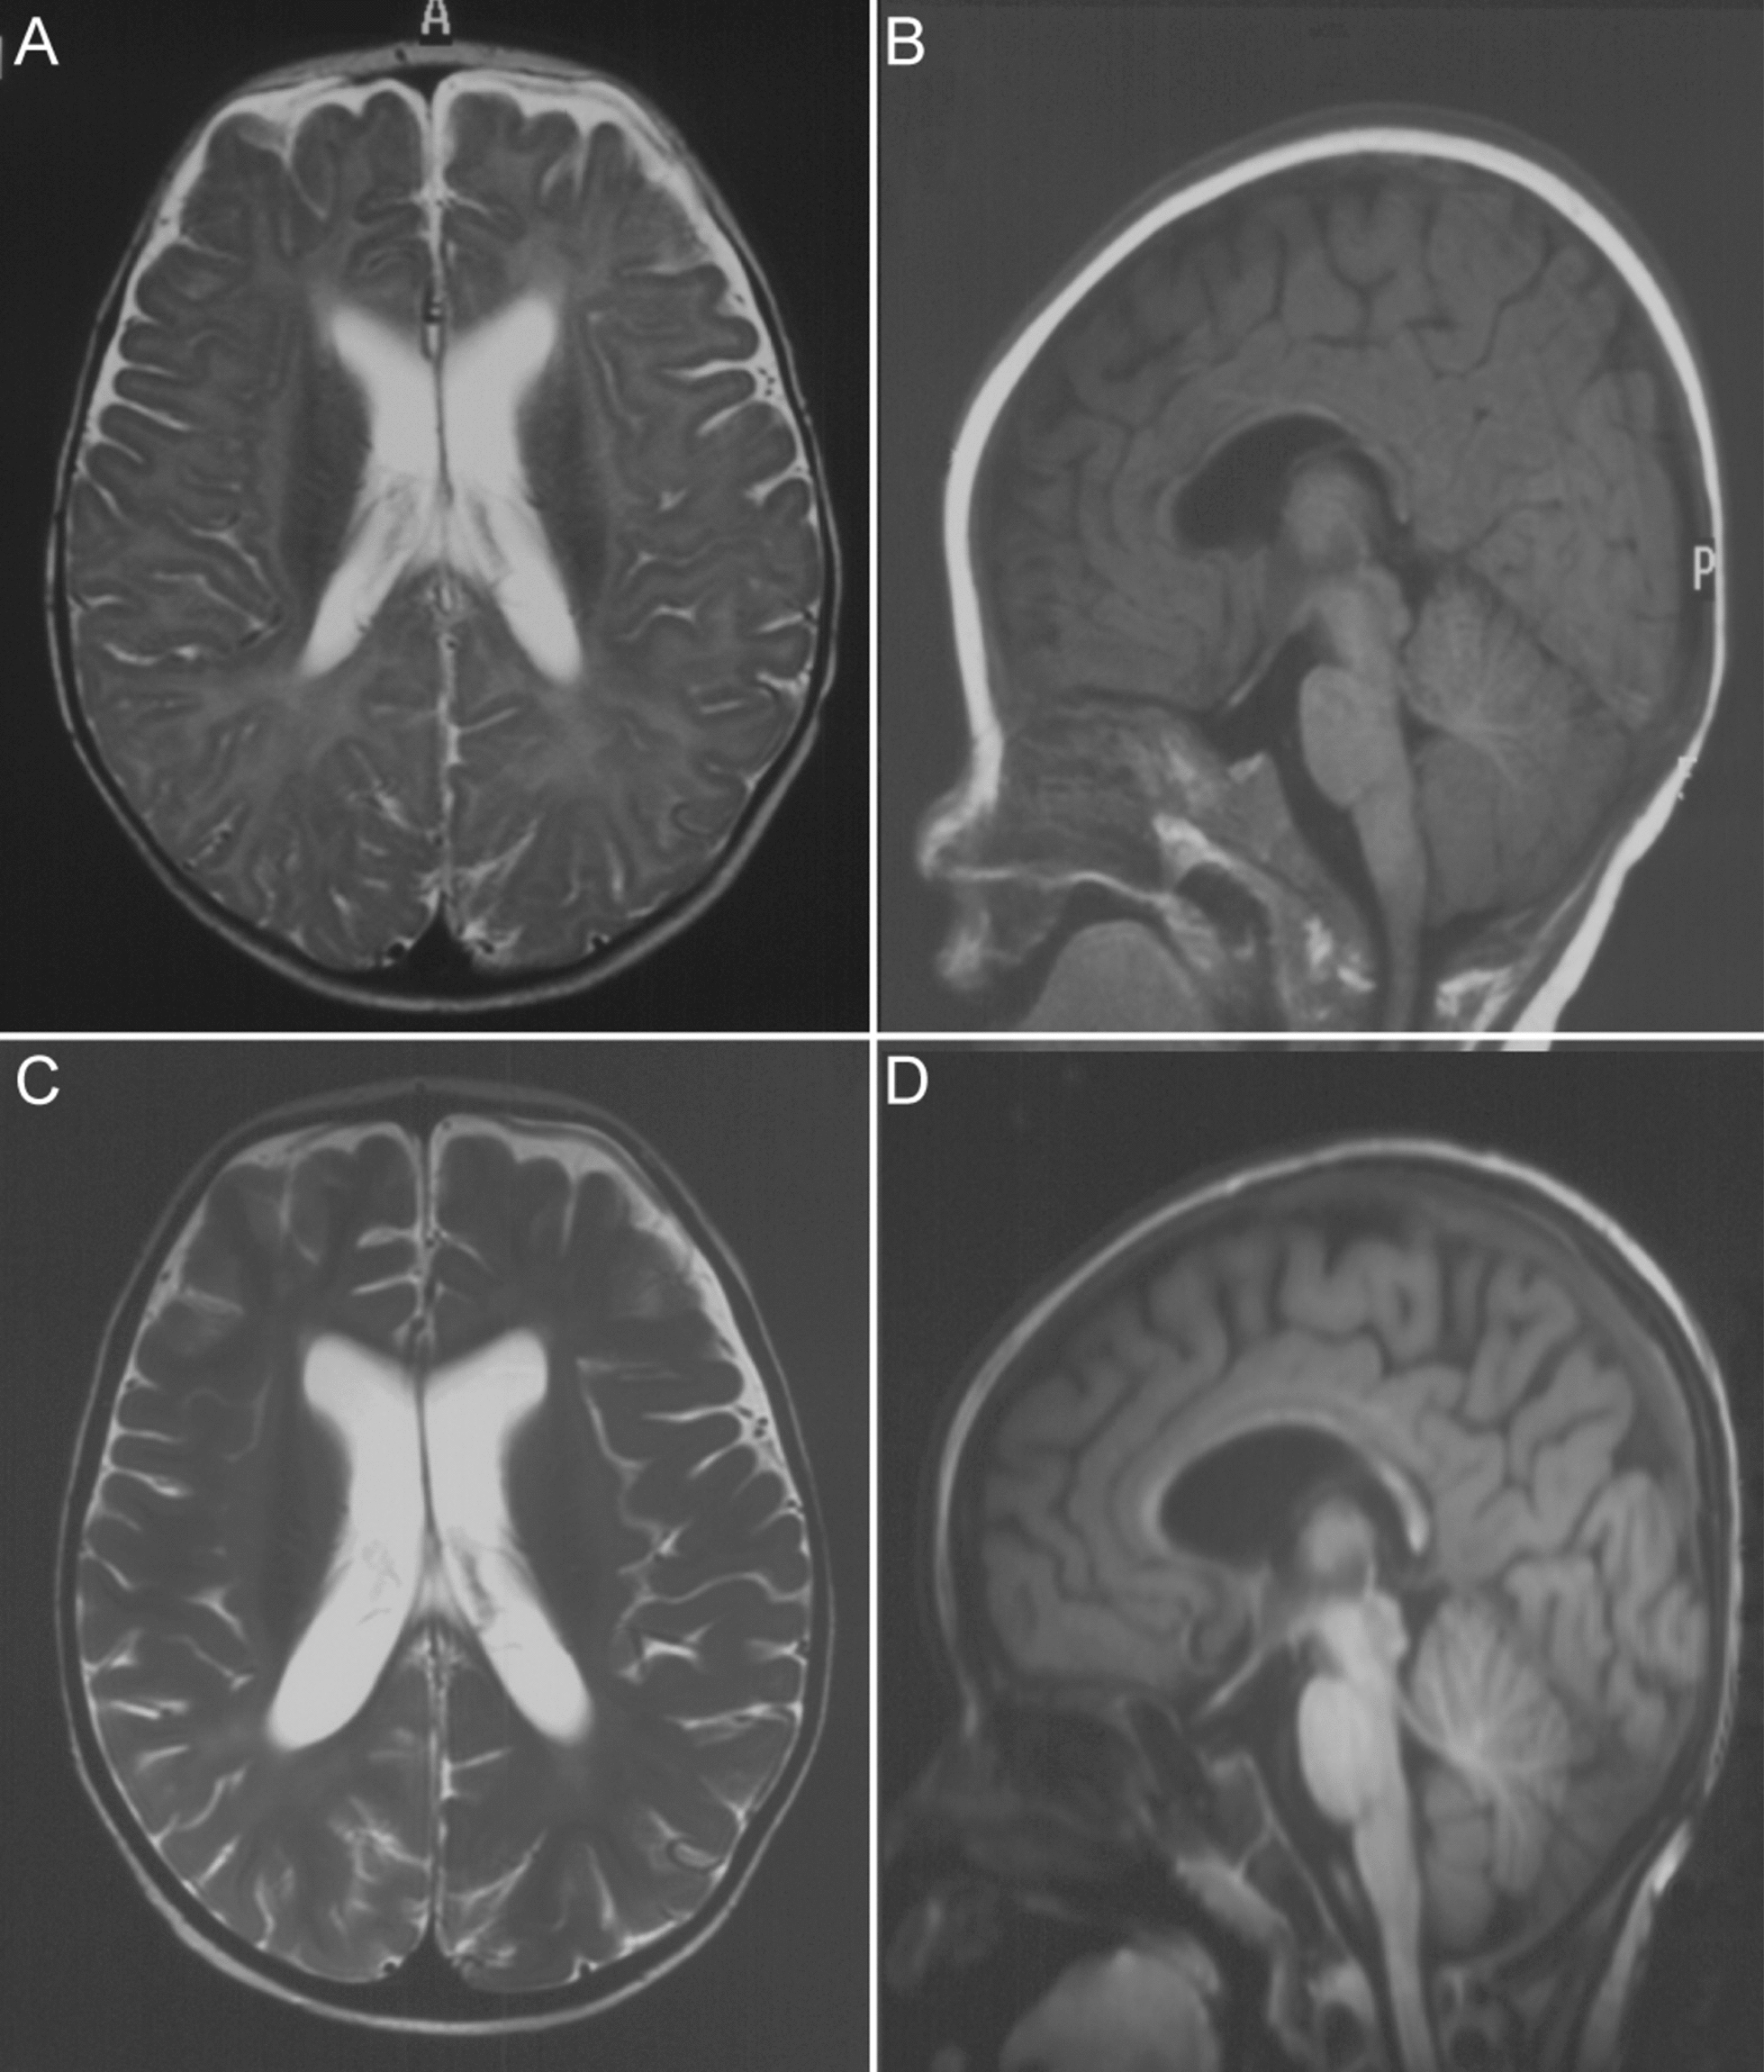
Cranial MR image of the patient with the *ATP6V0A1* variant. MRI performed at 4 months of age showed mild enlargement of the bilateral ventricles with a thin corpus callosum (A, B). MRI performed at 13 months of age showed progressive cerebral atrophy (C, D). A and C are T2-weighted images, and B and D are T1-weighted images
